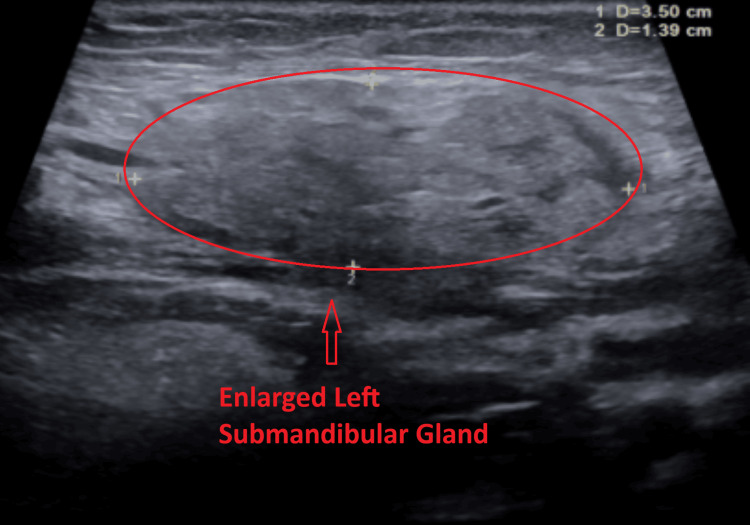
Ultrasound image of the transverse view of the left submandibular gland
